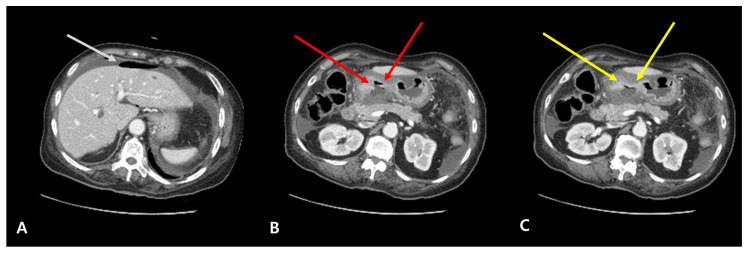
Abdominal computed tomography (CT) scans showing gastric cancer perforation. (A) Pneumoperitoneum with free intraperitoneal air (white arrow) is noted in the upper abdomen. (B) The perforation site is identified by a localized defect in the gastric wall (red arrows). (C) Marked, irregular thickening of the gastric wall (yellow arrows) is well-visualized, highly suggestive of the underlying gastric malignancy.
